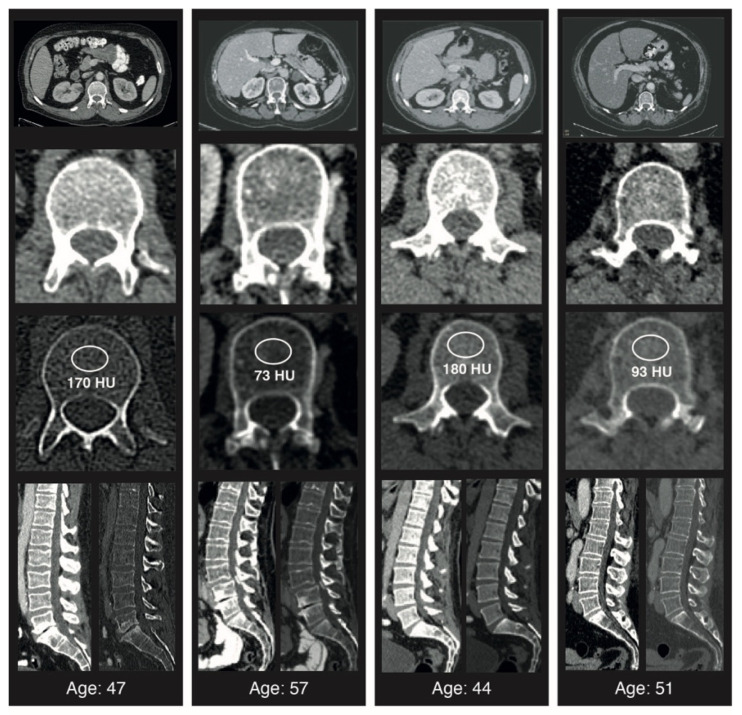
Examples of trabecular L1 attenuation assessment at CT. Transverse (axial) CT scans at the L1 level in adult patients of varying ages and with different average Hounsfield units at the time of diagnosis. Standard placement of the region of interest (ROI) for trabecular attenuation measurement and the mean Hounsfield unit value within the ROI are shown. Sagittal reconstructions with soft tissue and bone windows are shown.
